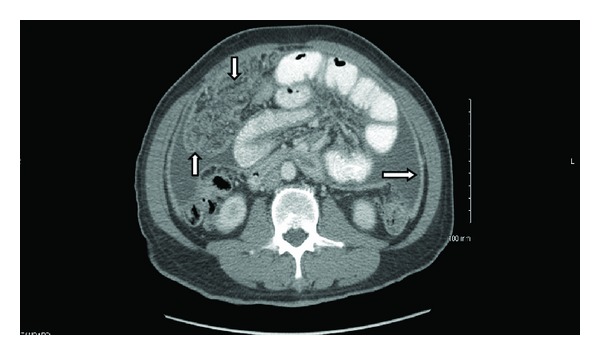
CT abdomen, moderate amount of abdominal, and pelvic ascites with diffuse thickening of peritoneal surfaces. Diffuse thickening also is seen throughout the omentum. Appearance is worrisome for peritoneal carcinomatosis.
